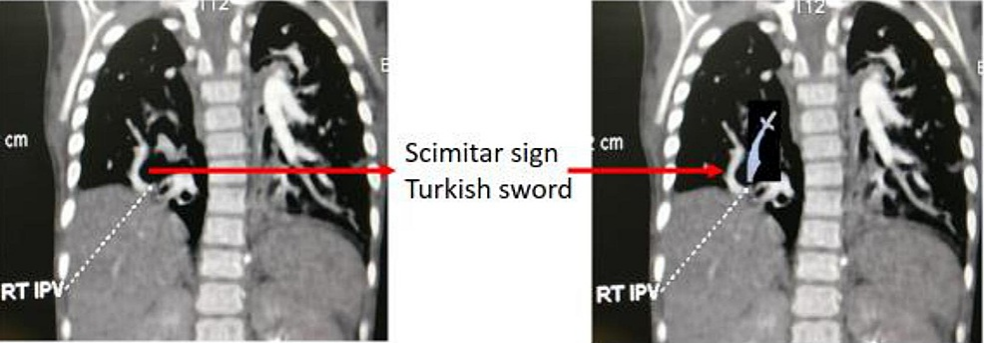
Turkish sword appearance in CT scan Image credits - Dr. Deepakkumar V. Mehta and Dr. Nirja Patel
